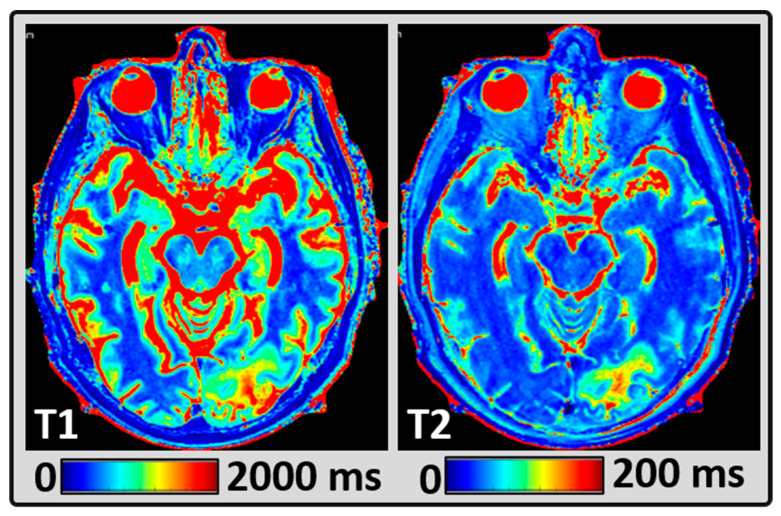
Representative MRI data from the same patient as Figure 2, showing quantitative images. (A and B) synthetically-generated T1 and T2 maps, respectively, from MAGiC.
